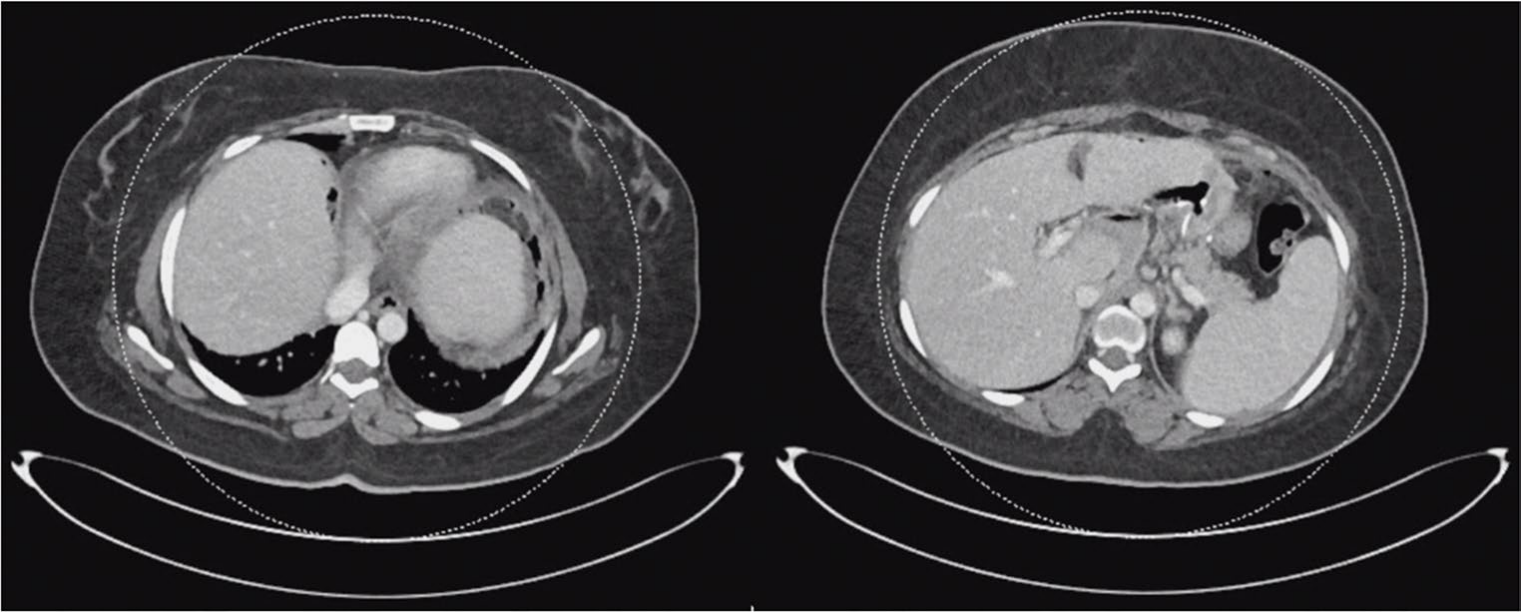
Axial contrast-enhanced computed tomography demonstrating free intraperitoneal air and perianastomotic fluid surrounding the gastrojejunal anastomosis, without contrast extravasation
